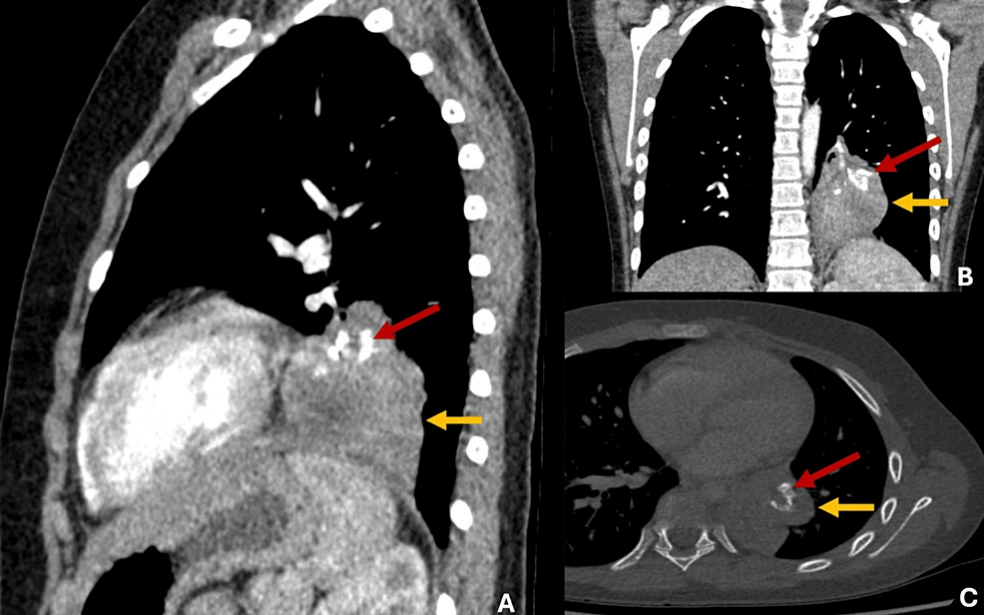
(A-C) Contrast-enhanced high-resolution chest CT reveals an intrapulmonary mass in the left inferior lobe measuring 45 mm x 51 mm. The mass exhibits irregular edges with soft tissue density (yellow arrows) and central calcifications (red arrows). Additionally, there was no evidence of feeders from the thoracic aorta.
